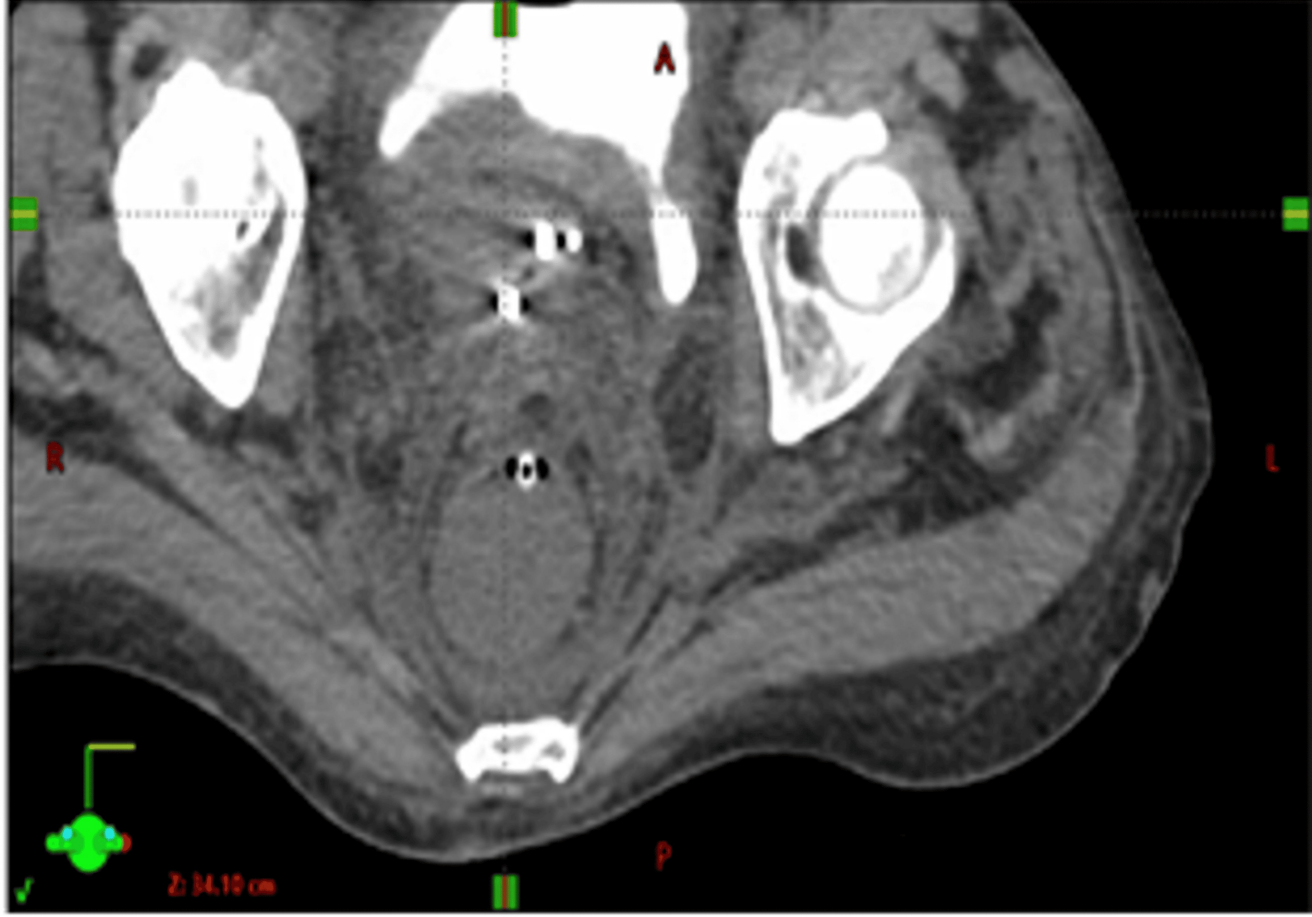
Coronal CT showing mini-ovoids
